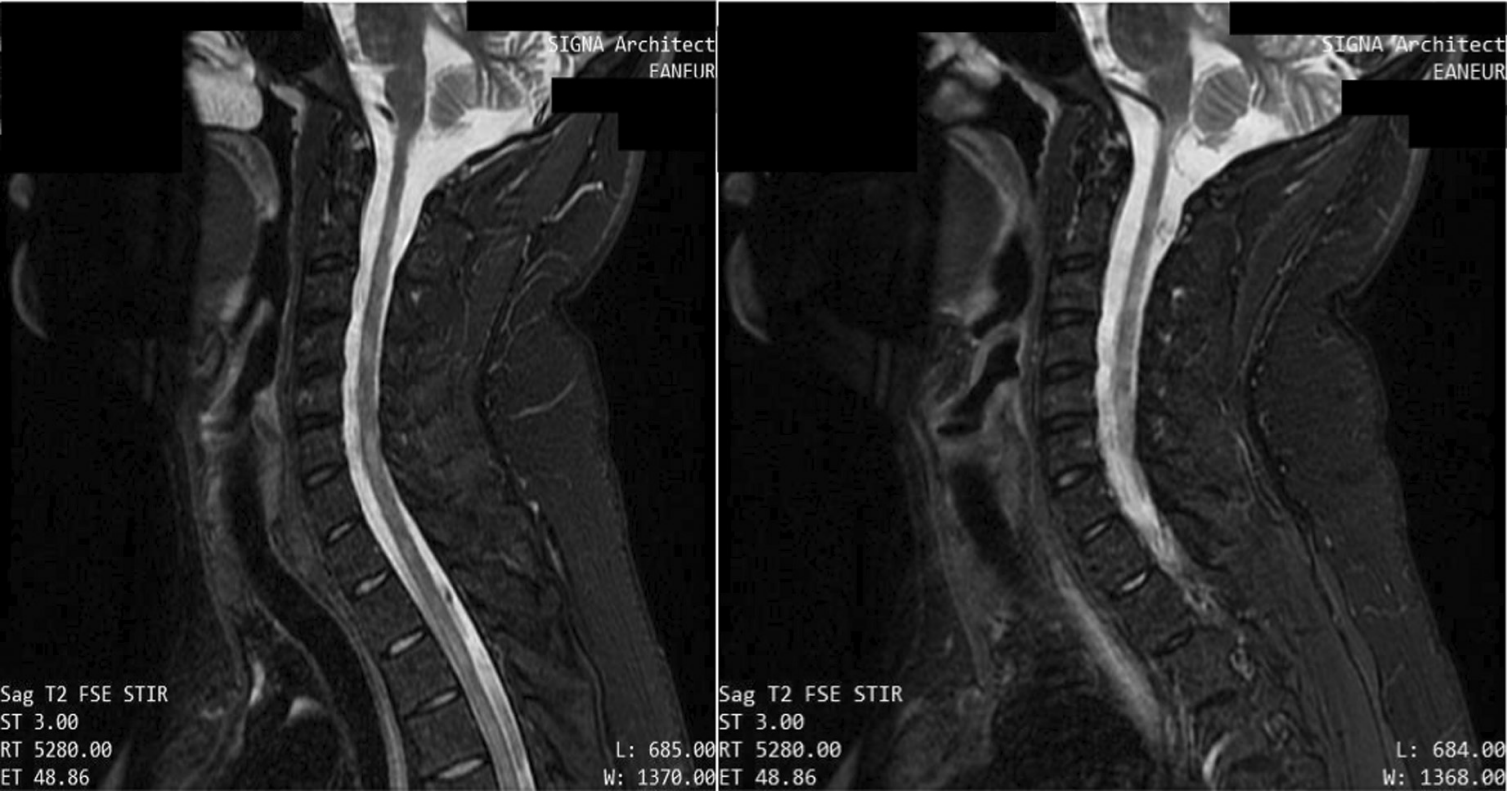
T2 STIR cervical MRI in April year X+2 showing hyperintense lesions.
